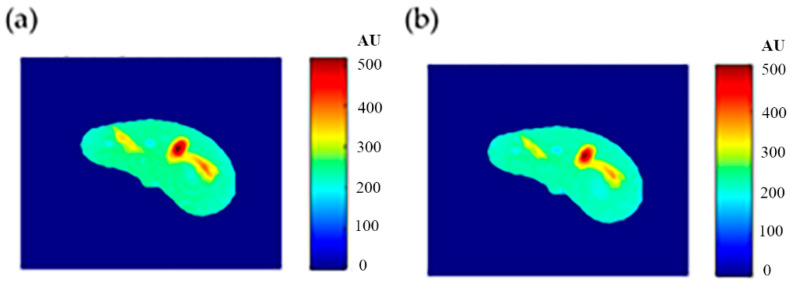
(a) The real MR image, and (b) the generated MR image using *T_E_* and *T_R_* of 20 ms and 200 ms, respectively.
